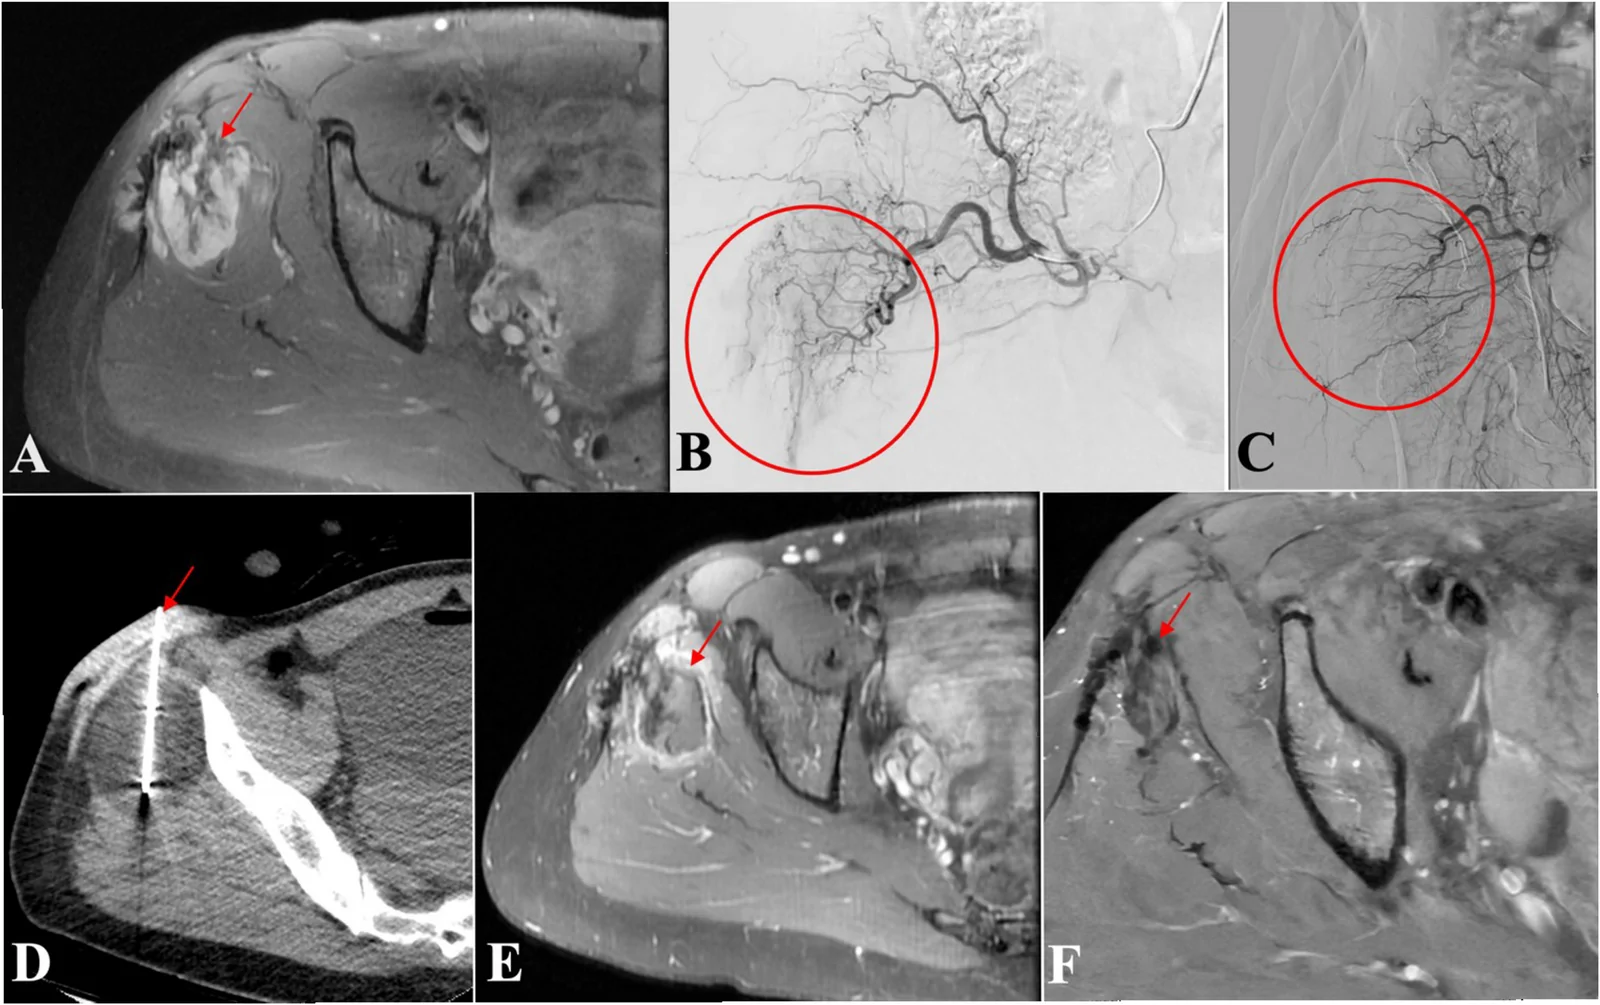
A 52-year-old woman with biopsy-proven desmoid-type fibromatosis of the right anterolateral gluteal region — refractory to four sessions of high-intensity focused ultrasound (HIFU) and sorafenib, with interval growth and right hip pain — underwent staged transarterial embolization and percutaneous cryoablation. A Pre-treatment axial T1-weighted post-contrast MRI showing an infiltrative enhancing lesion (arrow) measuring 10.7 × 4.2 × 3.4 cm in the right anterolateral gluteal musculature, primarily involving the proximal gluteus medius and iliotibial band, with mild interval growth from prior imaging. B Initial digital subtraction angiogram (DSA) of a selective branch of the right superior gluteal artery demonstrates hypervascular tumor blush consistent with desmoid tumor (circle). Embolization was subsequently performed using 500–700 μm Embosphere microspheres (Merit Medical, South Jordan, UT) until stasis C Digital subtraction angiogram (DSA) of the right internal iliac artery obtained after transarterial embolization demonstrates no residual tumor blush (circle) prior to cryoablation. D Intraprocedural axial CT image during percutaneous cryoablation, performed in the same session following embolization, demonstrates cryoprobe (arrow) within the lesion, with ice coverage extending beyond the margins of the targeted tumor. E Axial T1-weighted post-contrast MRI obtained approximately 3 months after cryoablation, demonstrates interval decrease in size and overall enhancement of the desmoid tumor (arrow), consistent with treatment response. Residual peripheral enhancement is consistent with posttreatment change, with no new nodular or mass-like enhancement. F Surveillance axial T1-weighted post-contrast MRI obtained approximately 3.5 years after cryoablation, demonstrating complete response (arrow), with no new suspicious lesions or significant interval growth, consistent with sustained treatment response. Case courtesy of Gina Landinez, MD
